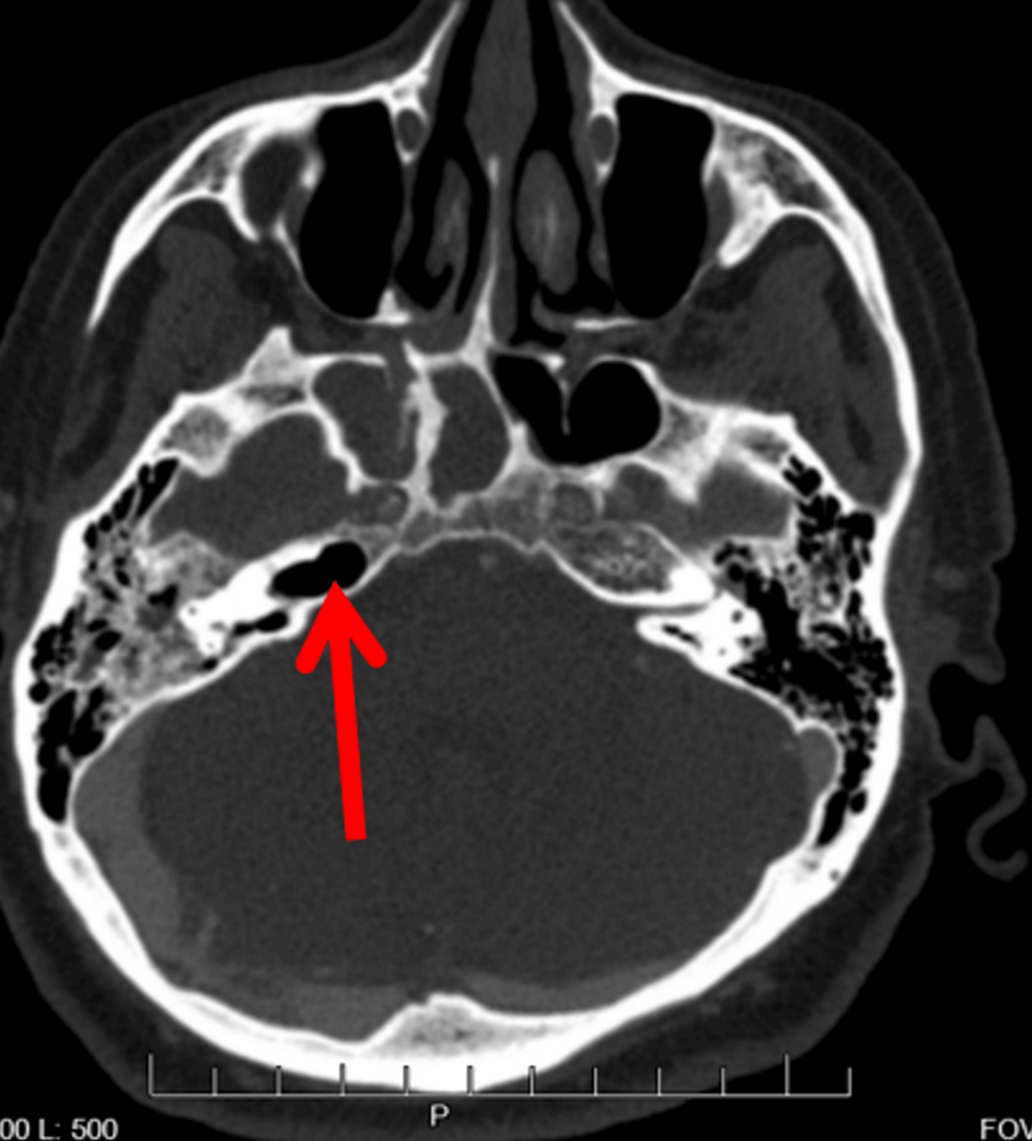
Initial CT showing osseous erosion of the right petrous temporal bone which also involves right carotid canal Red Arrow: Osseous Erosion
